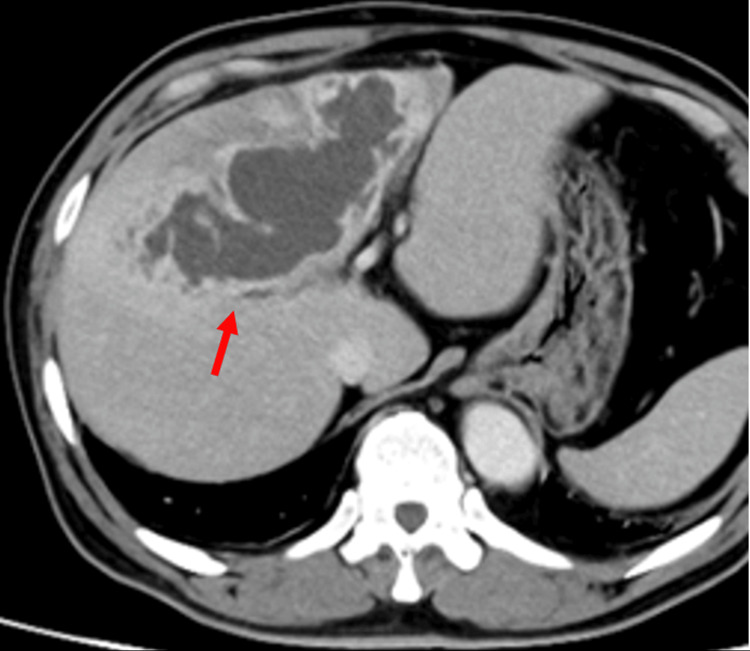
Contrast-enhanced CT shows a liver lesion. The red arrow points to a low-attenuation area in the left lobe of the liver with peripheral ring enhancement.
